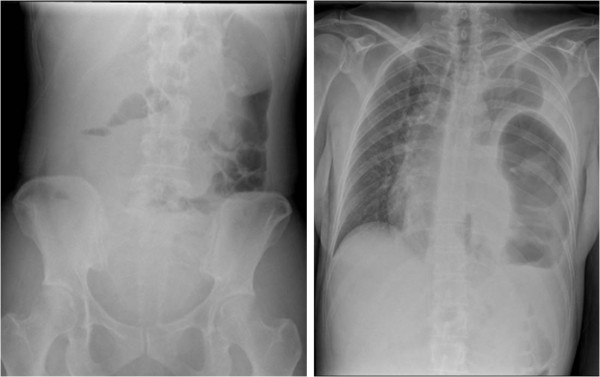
Abdominal radiograph 24 hours later shows a marked increase of bowel distension in left hemi thorax, resulting in a compression of left parenchyma. Descending and transverse colon is again distended. A mild fluid in the left pleural space is seen.
